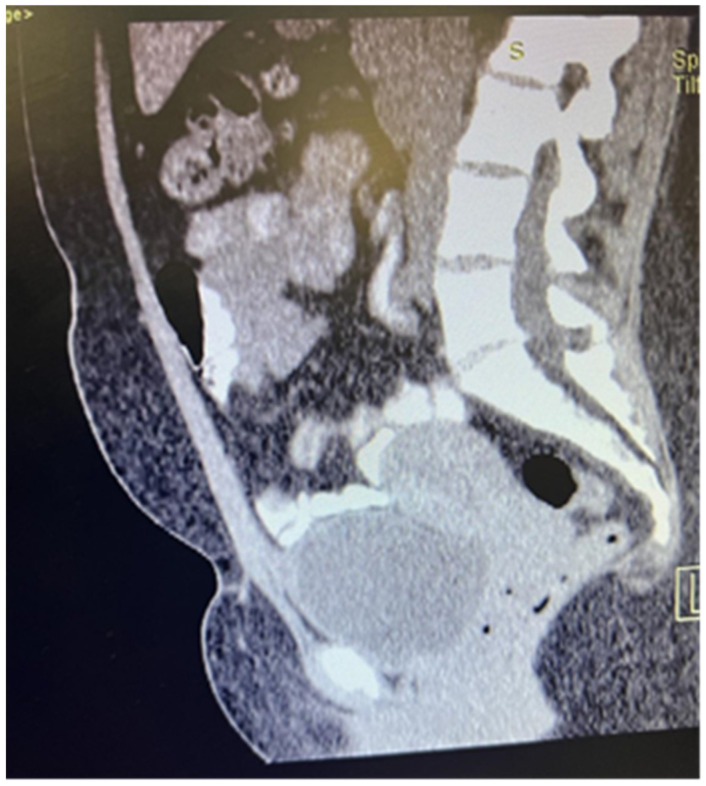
Computed tomography in sagittal section.
